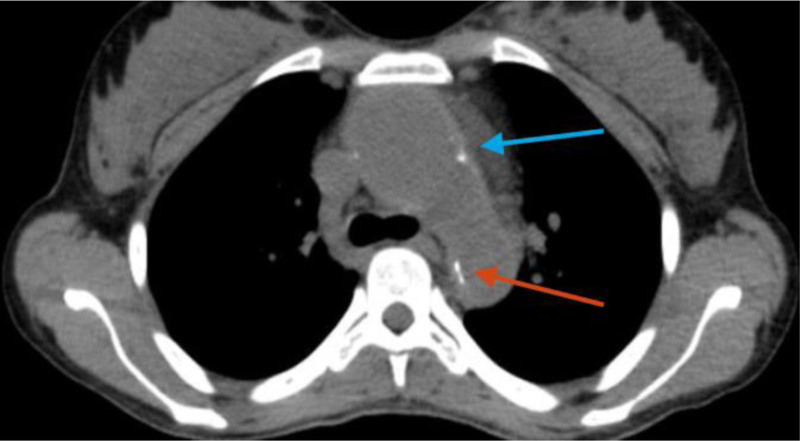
Pre-contrast CT scan: thickened aortic arch wall with luminal dilation, and calcification (blue arrow). Narrowed descending thoracic aorta with calcification (red arrow) was visualized. CT scan = computed tomography scan.
